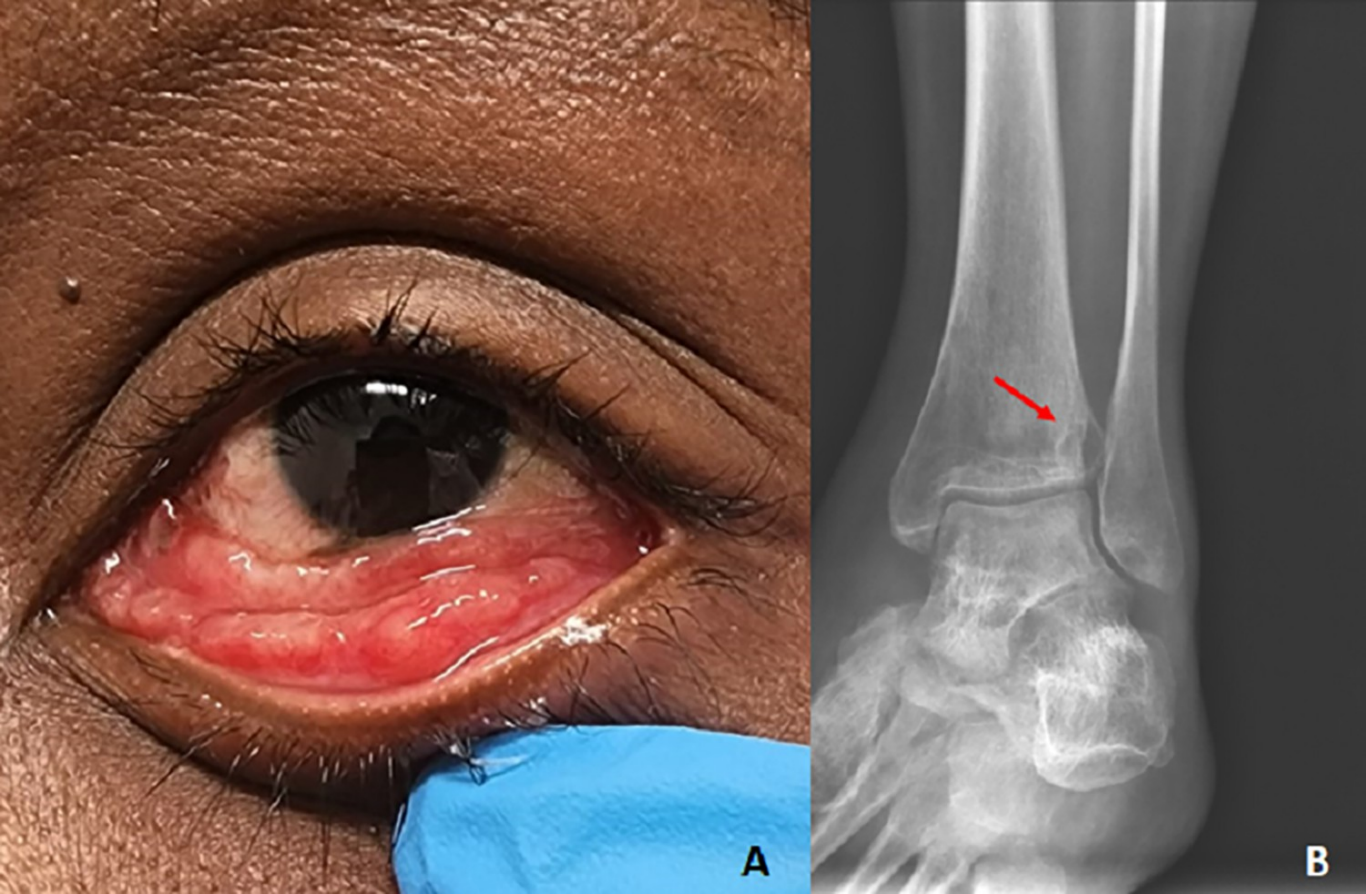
Pregnant women with extracutaneous/disseminated sporotrichosis. A) Case 52 with ocular sporotrichosis, presenting exuberant granulomatous conjunctivitis affecting the tarsal and bulbar conjunctiva of the left eye. B) Case 15 was a patient living with HIV with bone sporotrichosis lesion. Radiograph of the left foot and ankle showed a small lytic lesion in the distal tibia (red arrow), next to the lateral malleolus. Credit: Service of Image of Fiocruz, INI.
